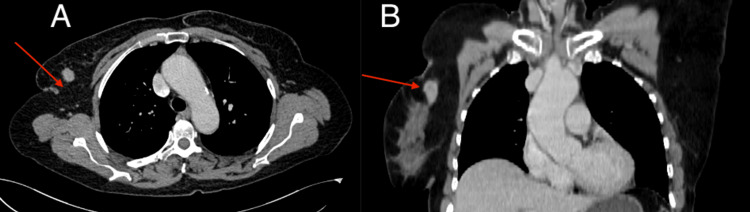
Chest CT scan with contrast injection revealing a right breast nodule in the upper outer quadrant (red arrows): (A) axial section and (B) frontal section
